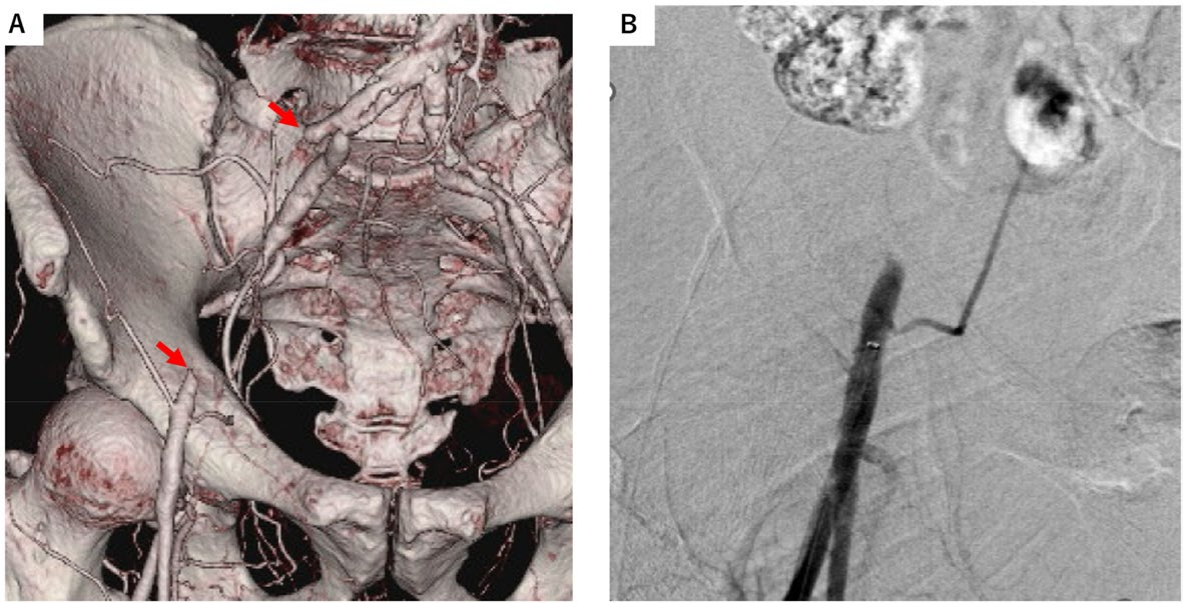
The findings of CTO lesion by enhanced 3-dimensional computed tomography (A) and initial angiography (B)
